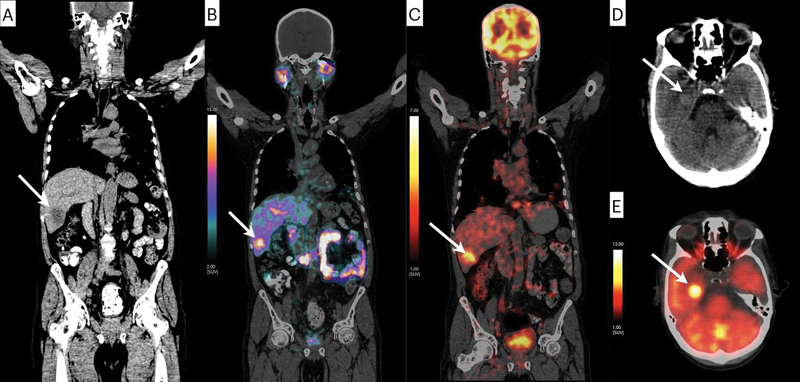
Coronal noncontrast CT (
